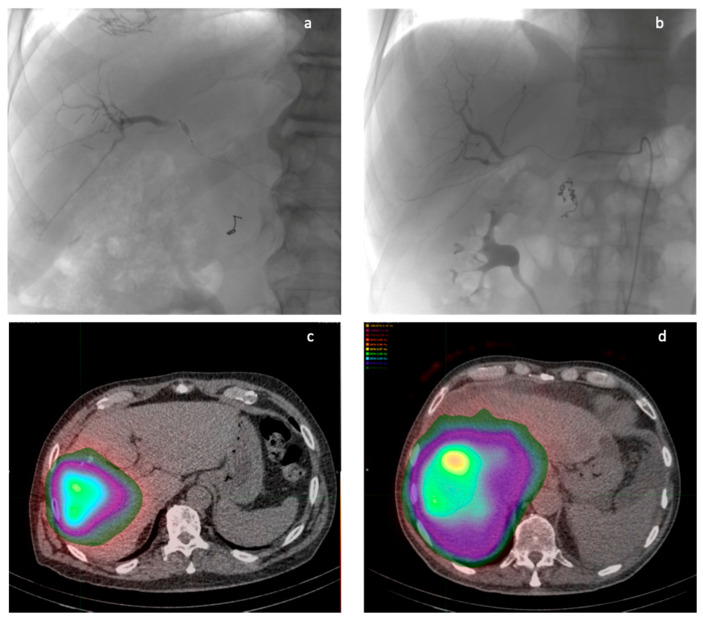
Comparison of two similar cases in terms of tumor burden, activity administered (1.88 GBq b-TARE, 1.90 GBq TARE), and position of the microcatheter’s tip before injection of 90Y microspheres. (a,b) show similar microcatheter tips in digital angiography, respectively, in the b-TARE patient and standard TARE patient; (c,d) show 90Y SPECT-CT fused image dose distribution, respectively, in the b-TARE patient and standard TARE patient.
